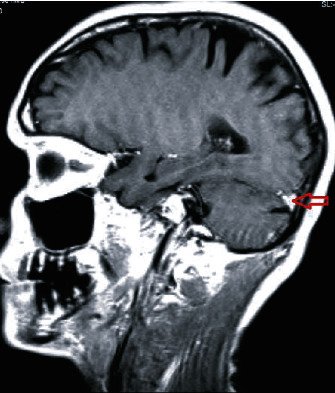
Patient MRI in sagittal view. Demonstrates calcifications in the supratentorial cerebellum.
